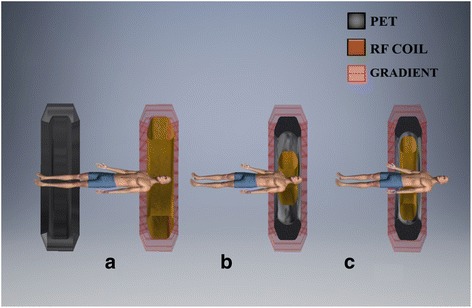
Shows the different configurations of PET/MRI (a) Sequential design, (b) Simultaneous PET insert MRI scanner and (c) Simultaneous fully integrated system
